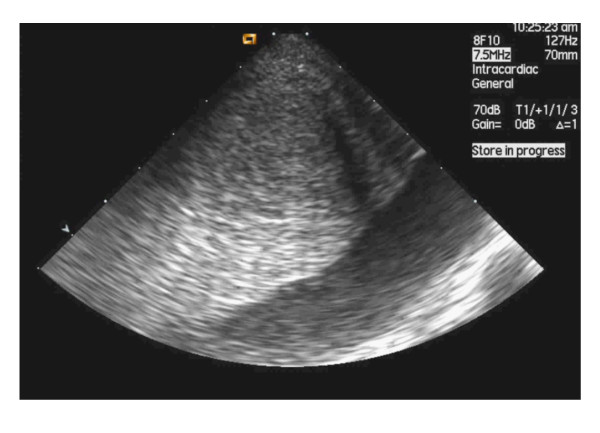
Ascites in moderate quantity was visualized from the hepatic vein by intracardiac ultrasound technique. The presence of an irregular hepatic border suggests nodular reorganization of the hepatic parenchyma as seen in cirrhosis.
